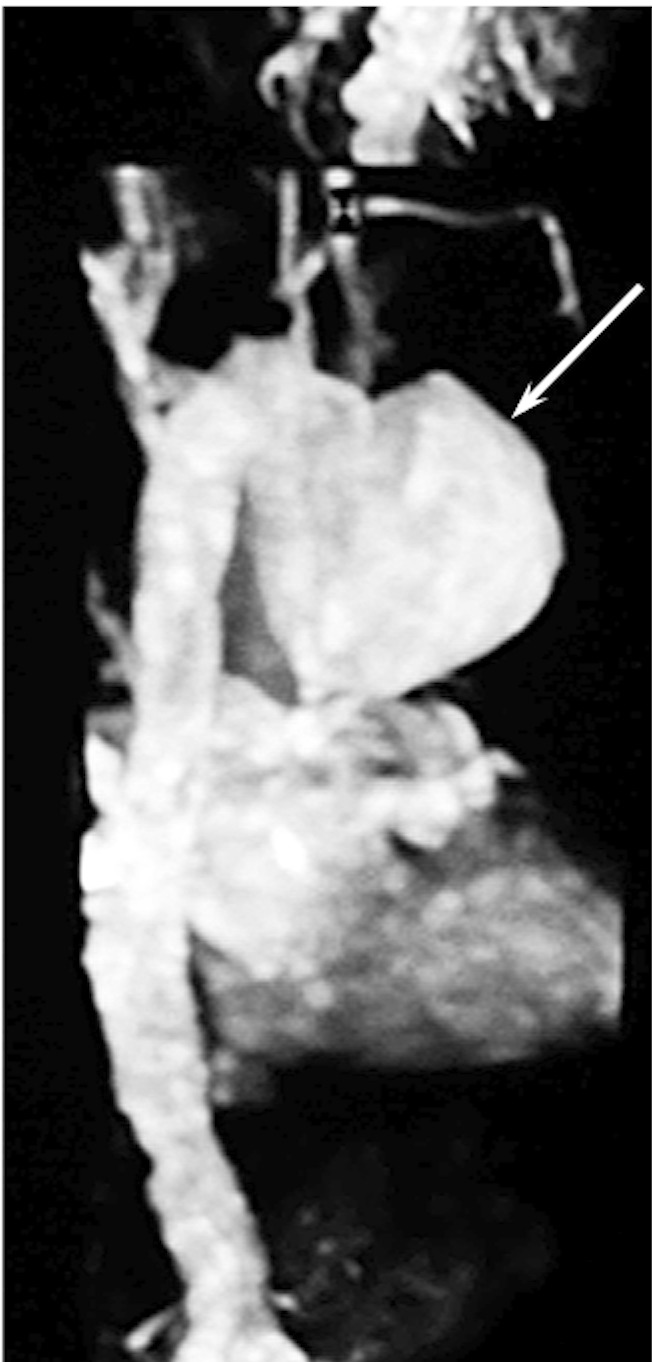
Giant saccular aortic arch aneurysm (white arrow) diagnosed by magnetic resonance imaging (MRI).
